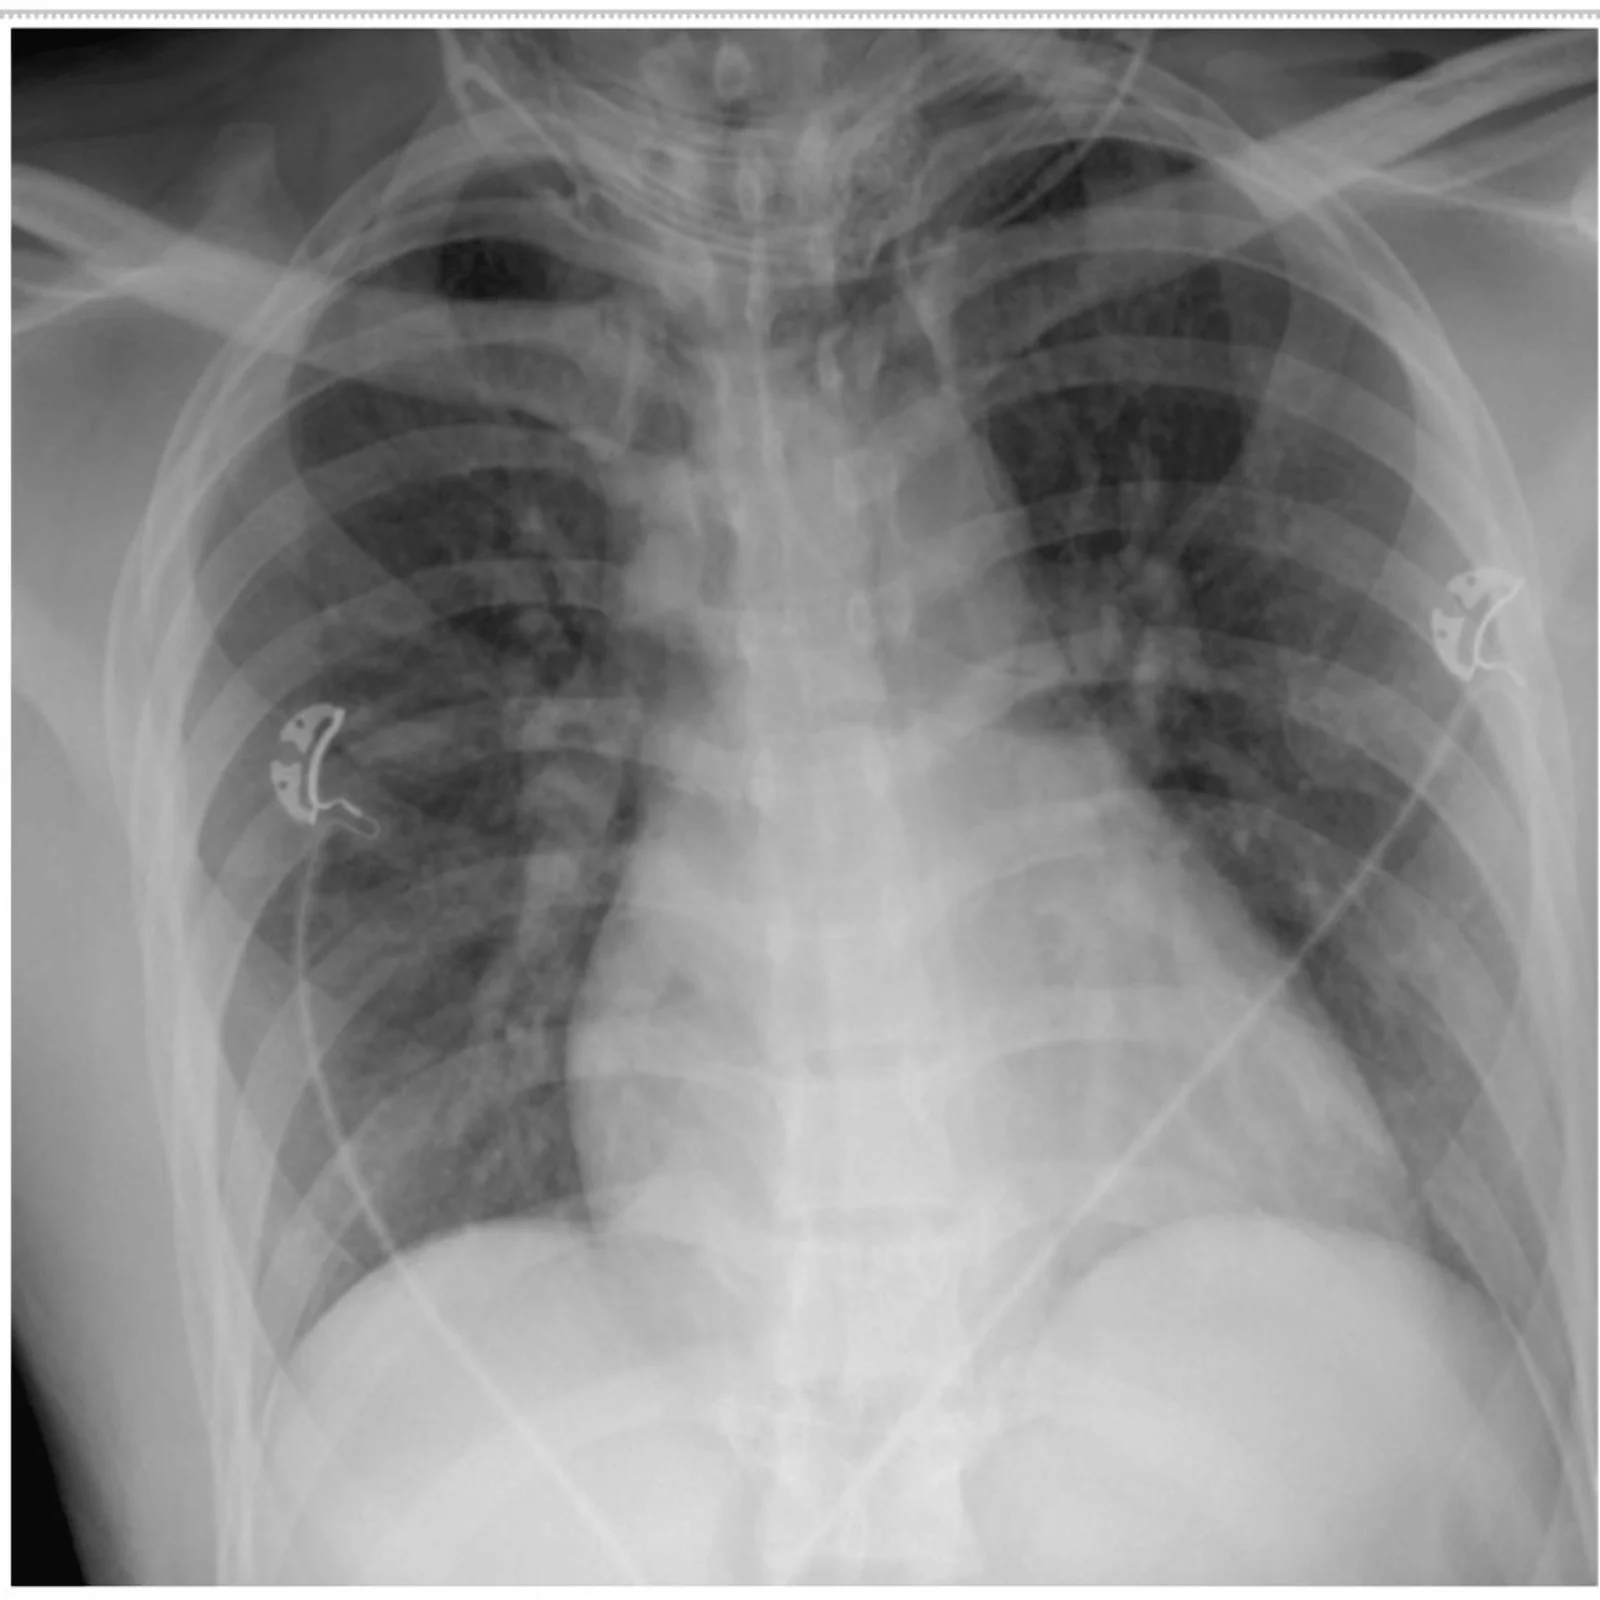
Chest X-ray with no acute cardiopulmonary process. Airway is midline. Cardiac silhouette within normal limits. No sizeable pleural effusion or pneumothorax. Cervical collar in place.
